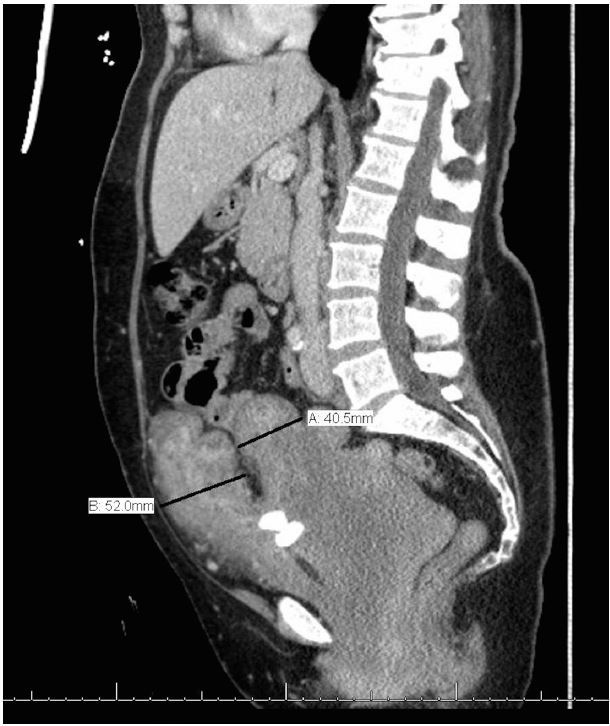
Computed tomography of enlarged, nondistended urinary bladder with diffusely thickened wall shown in sagittal view after placement of a urinary catheter.
